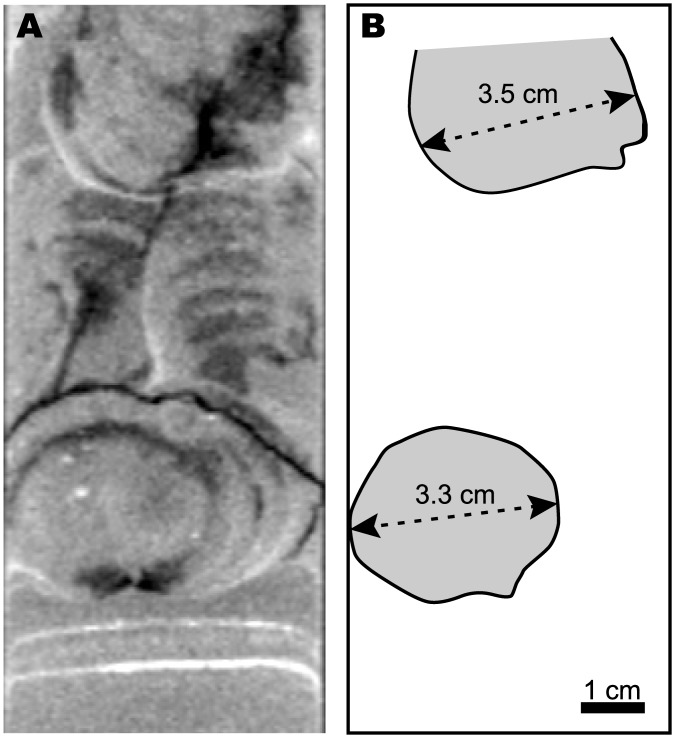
Close-up view of the burrows produced by *Echinocardium cordatum*. (A) CT image of the upper part of core F-2. (B) Sketch of (A). The transverse width of the *E*. *cordatum* burrows is ~3 cm.
